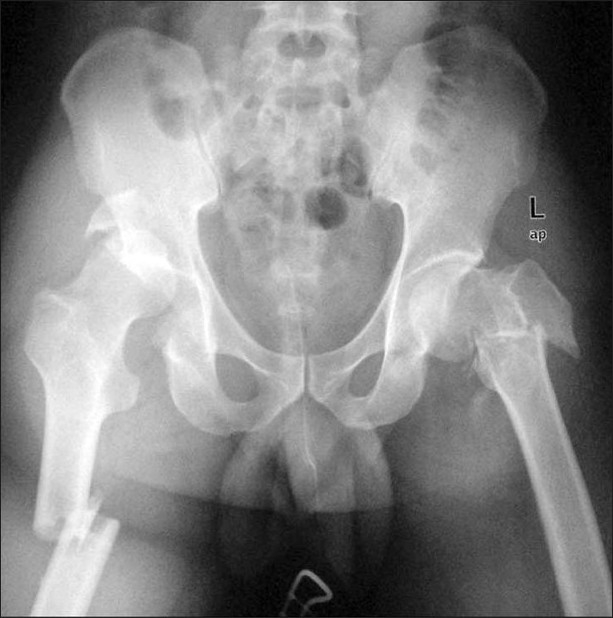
Radiograph of the pelvis including both hips and thighs (an anteroposterior view) at presentation showing pertrochanteric fracture of the left femur with an ipsilateral femoral neck fracture and posterior dislocation of the right hip with a posterior acetabular wall fracture with an ipsilateral right femoral shaft fracture
